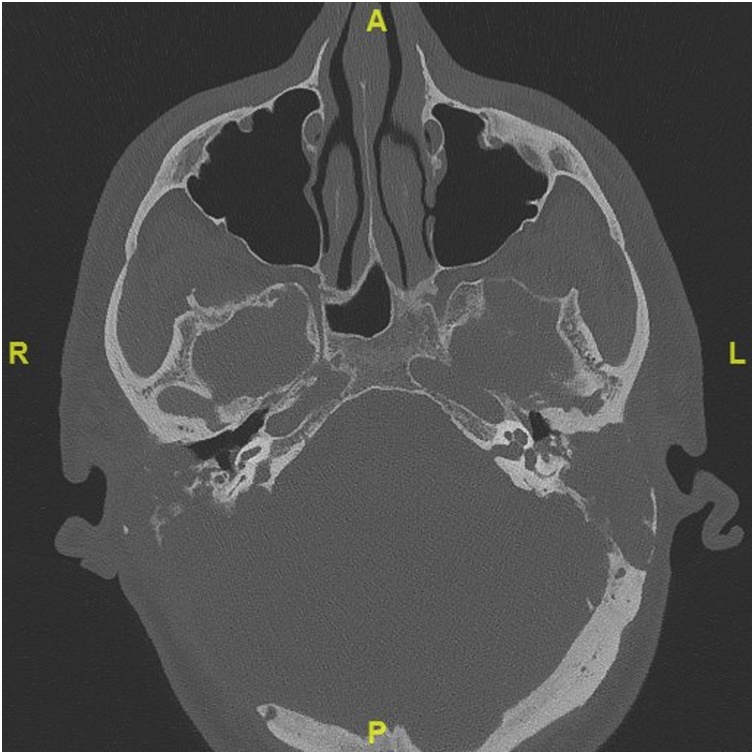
CT scan hypodense, soft tissue, posterior fossa mass destroying the right petrous bone and the adjacent occipital and temporal bones. A similar lesion involving the left mastoid portion of the petrous bone with a small tissue component was seen in the posterior fossa on the left side.
